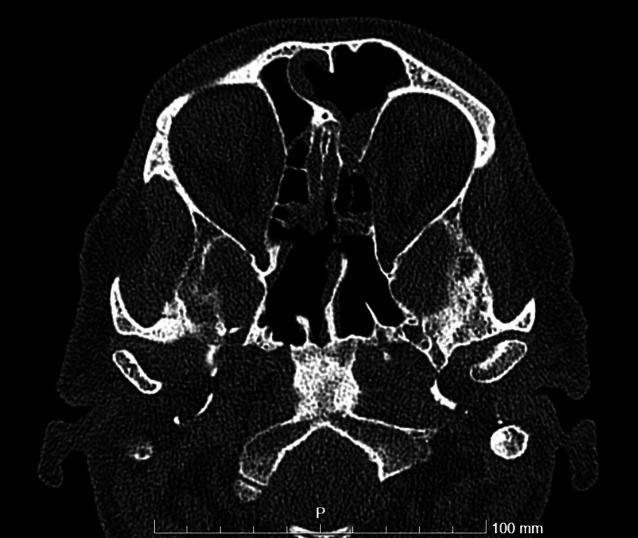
CT-NNH, transversale Schnittführung: postoperativ frei belüftete Sinus sphenoidales beidseits
